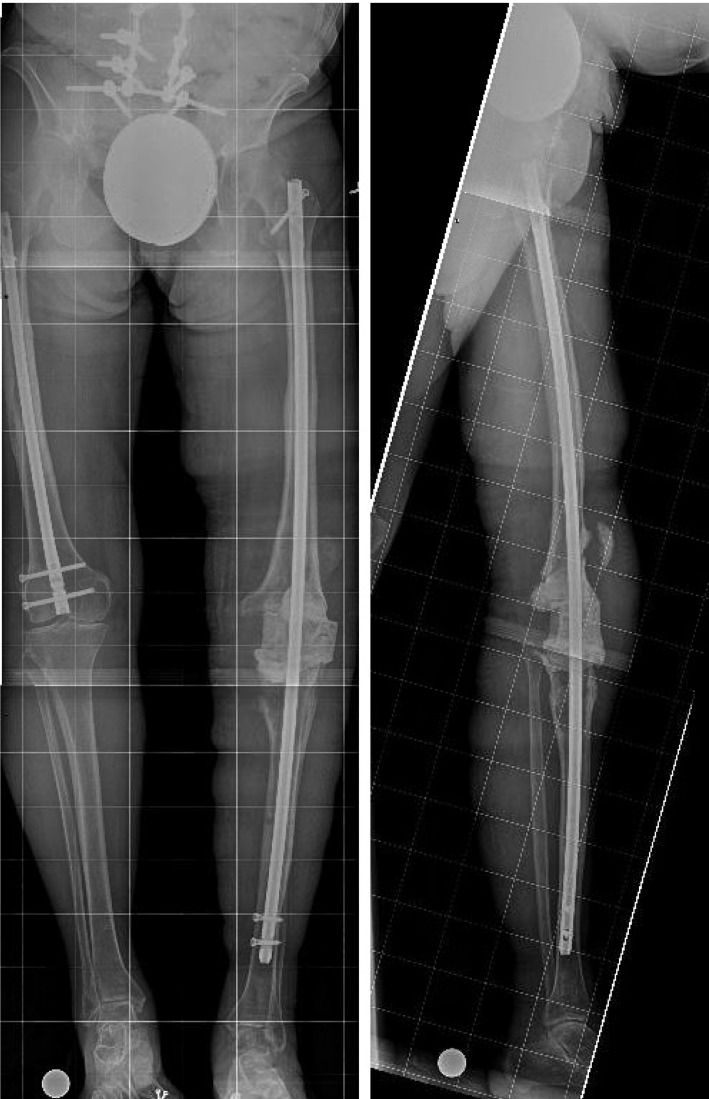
1/20/2015. AP (A) and Lateral (B) radiographs demonstrating a locked lower extremity arthrodesis nail through a static antibiotic cement block.
